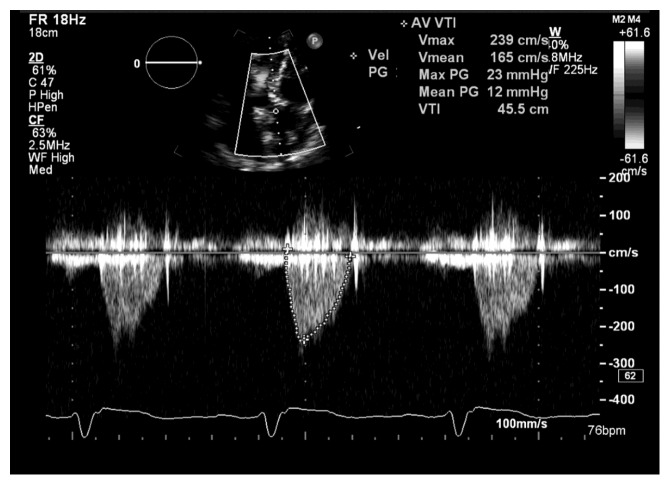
Transaortic Doppler at 30 days after TAVI reveals mean systolic gradient of 12 mmHg and no aortic insufficiency. TAVI = transcatheter aortic valve replacement.
